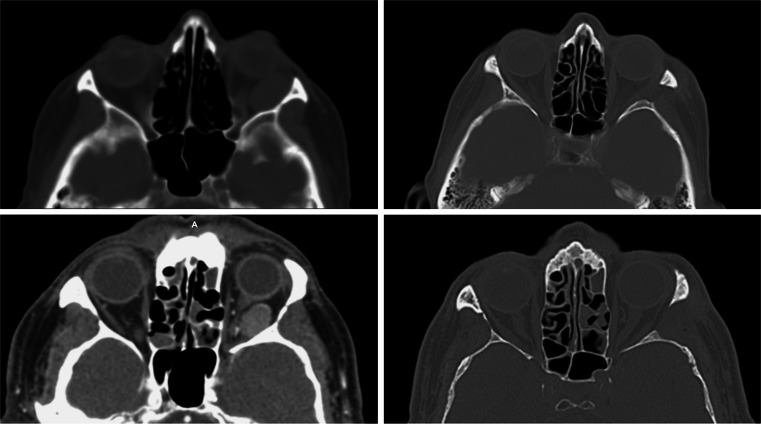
Preoperative (left) and postoperative (right) axial CT scans of two patients with intraorbital pathologies handled with endoscopic lateral orbitotomy: epithelioid hemangioma (higher) and cavernous angioma (beneath)
